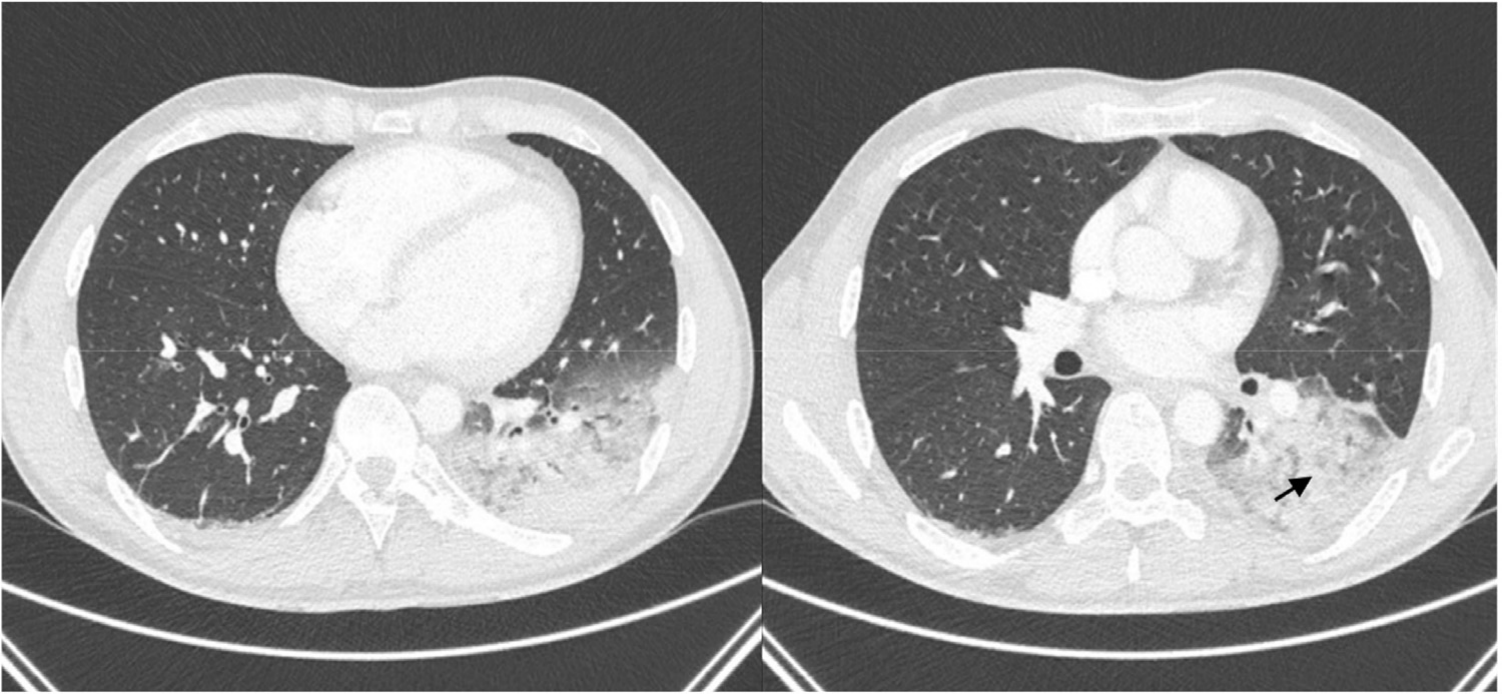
Chest computed tomography scan showed subpleural ground-glass opacities (black arrow) with a basal distribution in the left lower lobe and alveolar consolidations.
